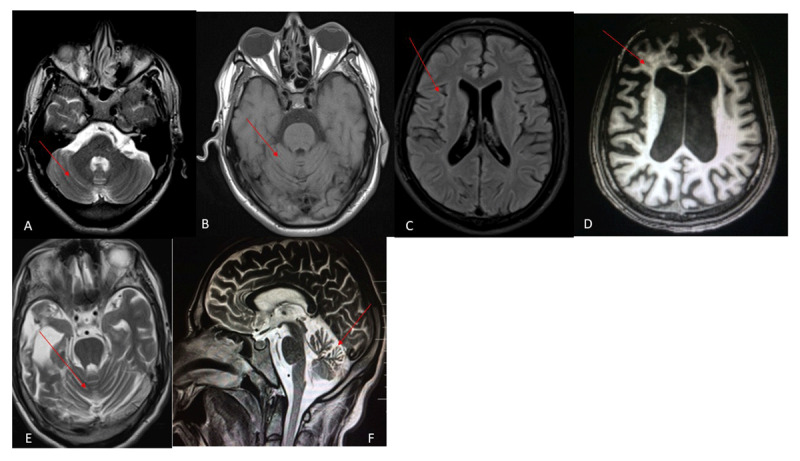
Brain MRI of patients with adult-onset NPC; Patient no 2 (A) Sagittal T2-weighted image showing cerebellar atrophy (red arrow); Patient no 3 (B, C)- (B) Axial T1-weighted image showing frontal atrophy with prominent ex-vacuo dilation of lateral ventricles (red arrow); (C) Axial T2-weighted image showing bilateral temporal atrophy and cerebellar atrophy (red arrow); Patient no 4 (D-F); (D) Axial T2-weighted image showing cerebellar atrophy (red arrow); (E) Axial T1-weighted image showing cerebellar atrophy (red arrow); (F) Axial FLAIR image showing cortical atrophy (red arrow).
